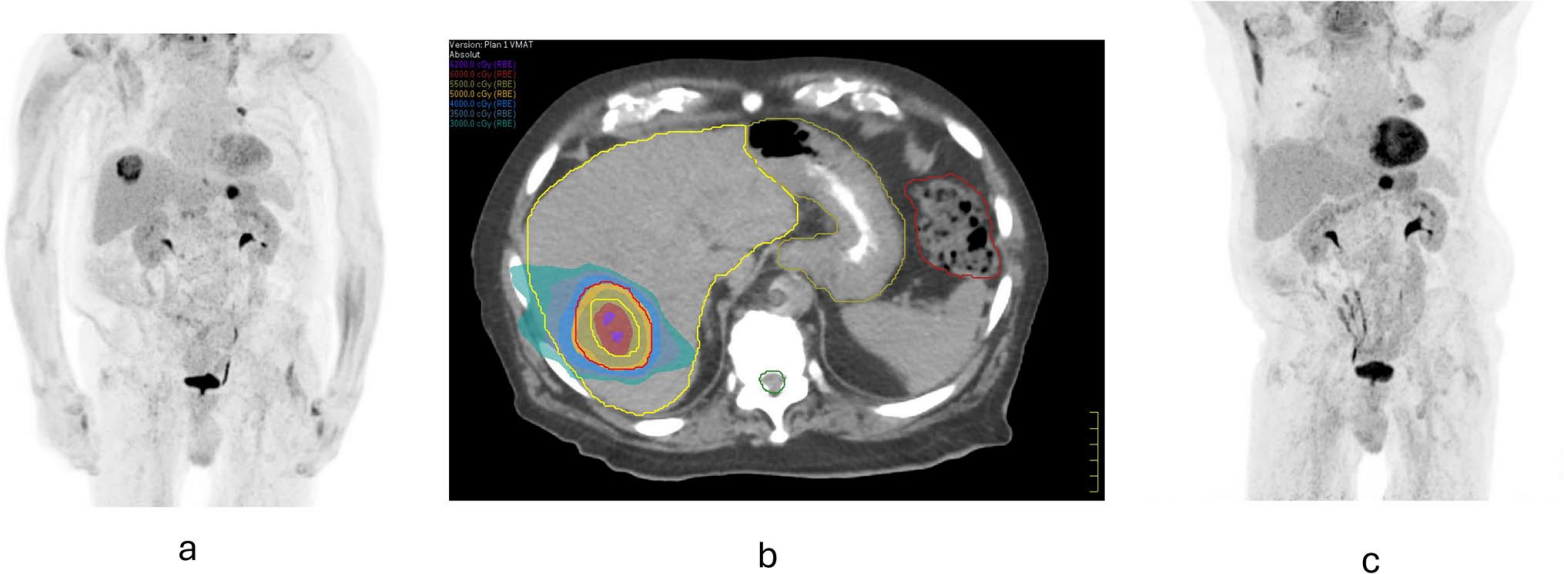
a Pre-treatment PET-CT demonstrating hypermetabolic activity in a liver metastasis segment. b Axial planning CT scan with radiation treatment plan showing the dose distribution over the target lesion in the liver. color wash lines represent different isodose levels, with adequate sparing of adjacent organs at risk (OARs). c Three months Post-treatment PET-CT showing the complete metabolic response of the lesion following SBRT, with reduced hypermetabolic activity
